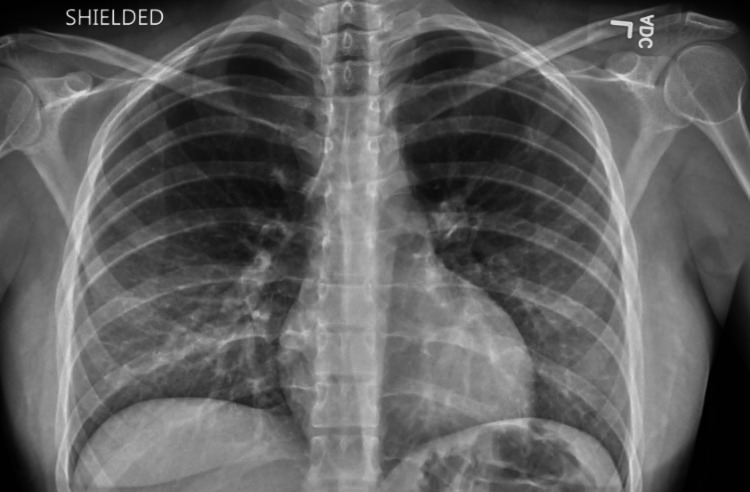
Antero-posterior chest radiograph showing minimal bilateral pleural effusion and pulmonary edema.
